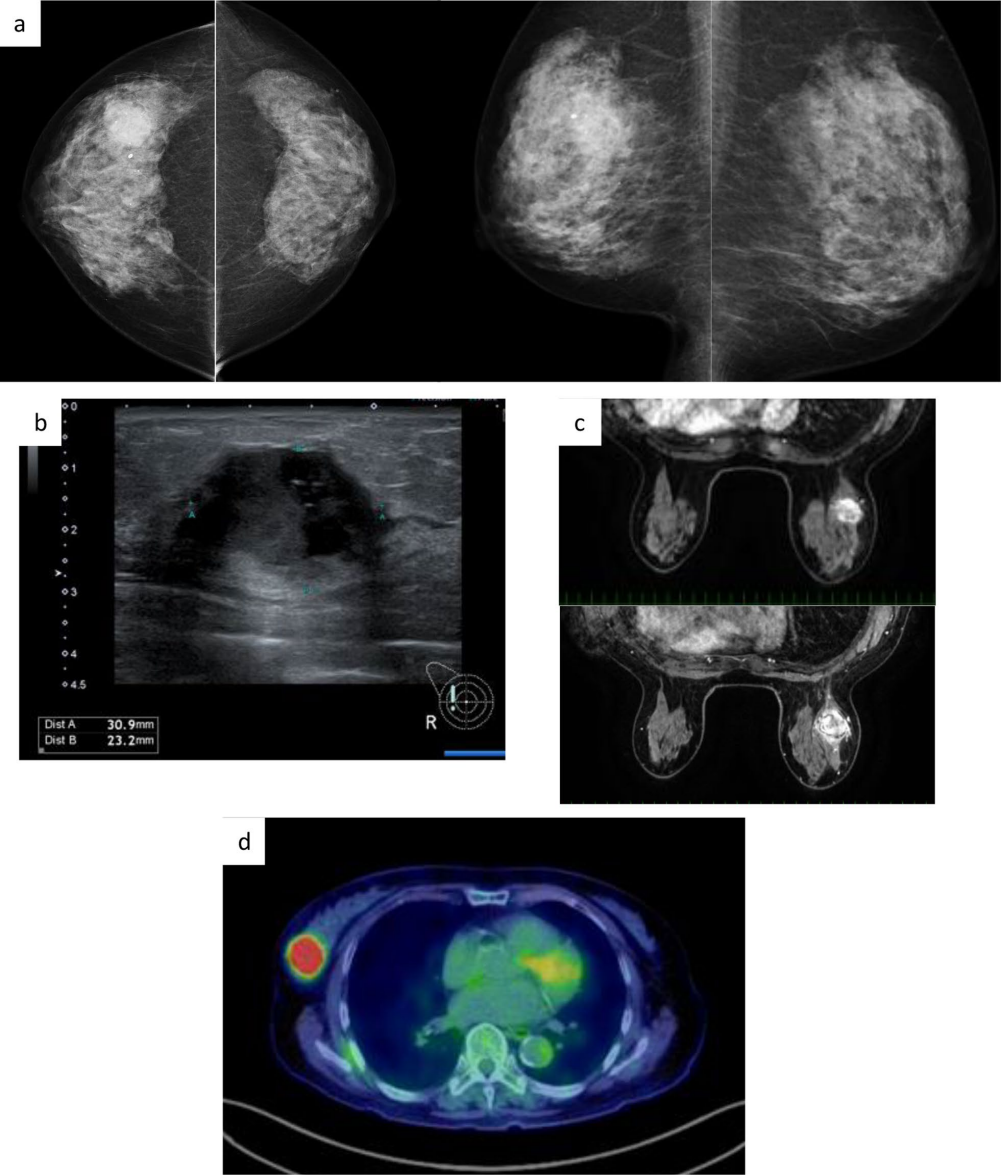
Images of the patient at the first visit. a Mammography: high-density mass shadow with obscure margin in the outside area of the craniocaudal outside and middle area of the mediolateral oblique. b Images of ultrasonography. Irregular nodule 31 × 23 mm in size. c Magnetic resonance imaging. Upper: early phase; Lower: late phase. Round mass with high intensity in T2 mode with rapid wash-out sign. d Fluorodeoxyglucose (FDG)–positron emission tomography. High concentration of FDG in the upper-outer region of the breast
